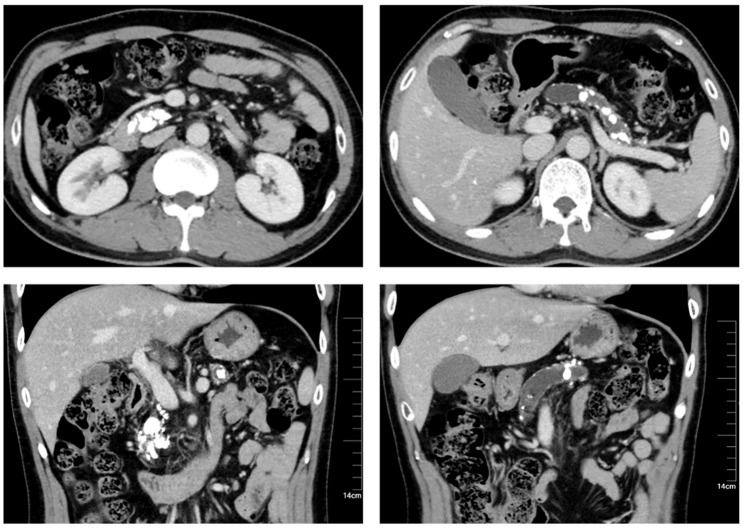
Computed tomography scan of the abdomen showing multiple pancreatic stone with pancreatic ductal dilatation and parenchymal atrophy of the pancreas
